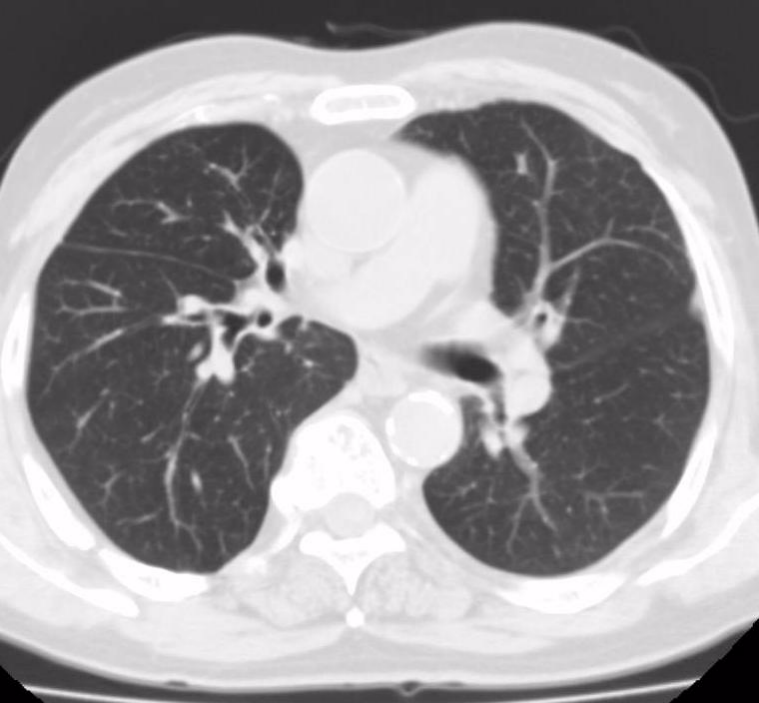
CT images before SBRT (Pt. No. 7).
